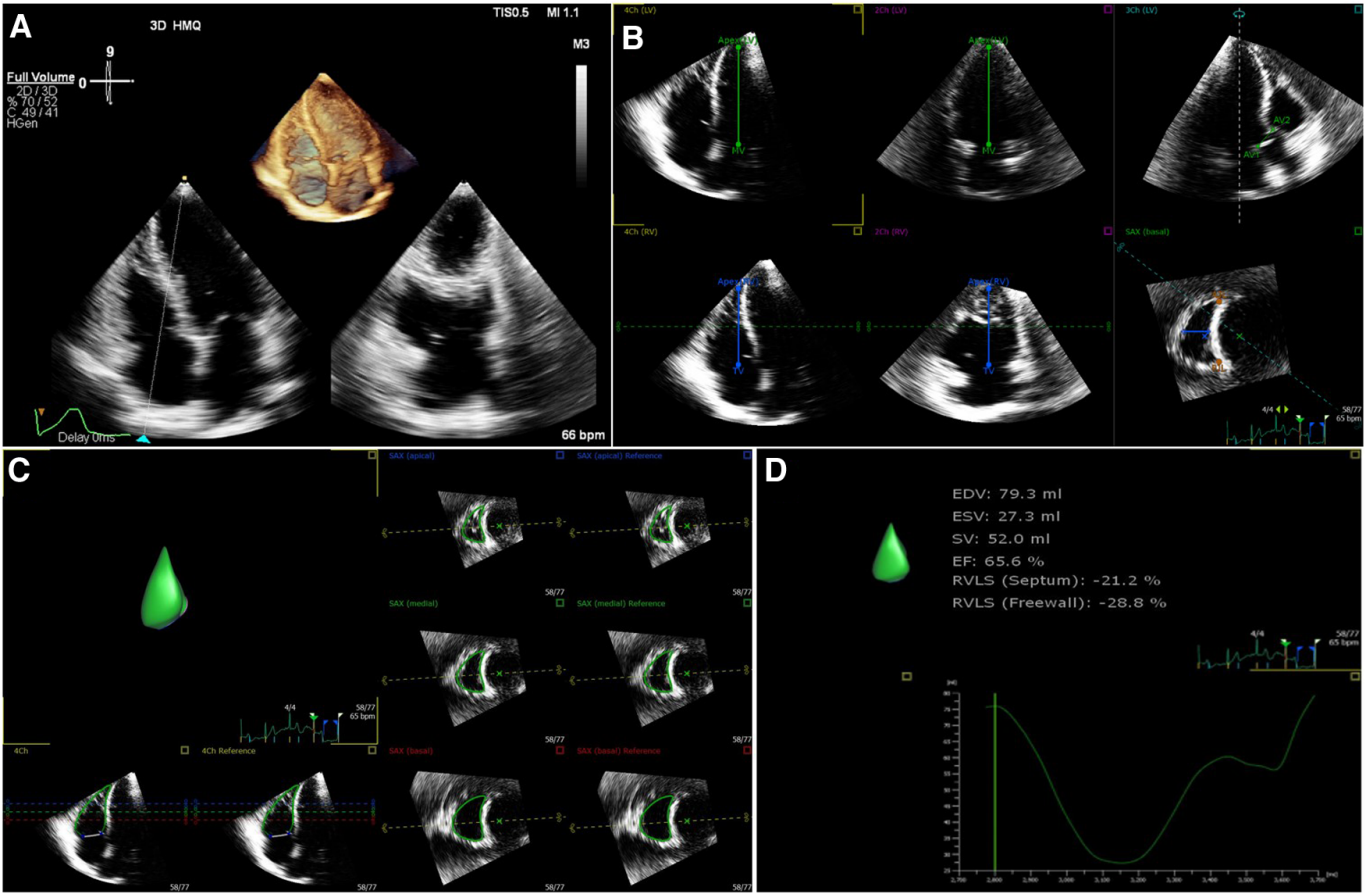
Rv three-dimensional image. (A) Three-dimensional image of RV-focused apical 4-chamber view. (B) Reference points setting. (C) RV endocardial border identification and tracking at end-systole and end-diastole. (D) Longitudinal strain of RV free wall and septum. RV, right ventricular.
